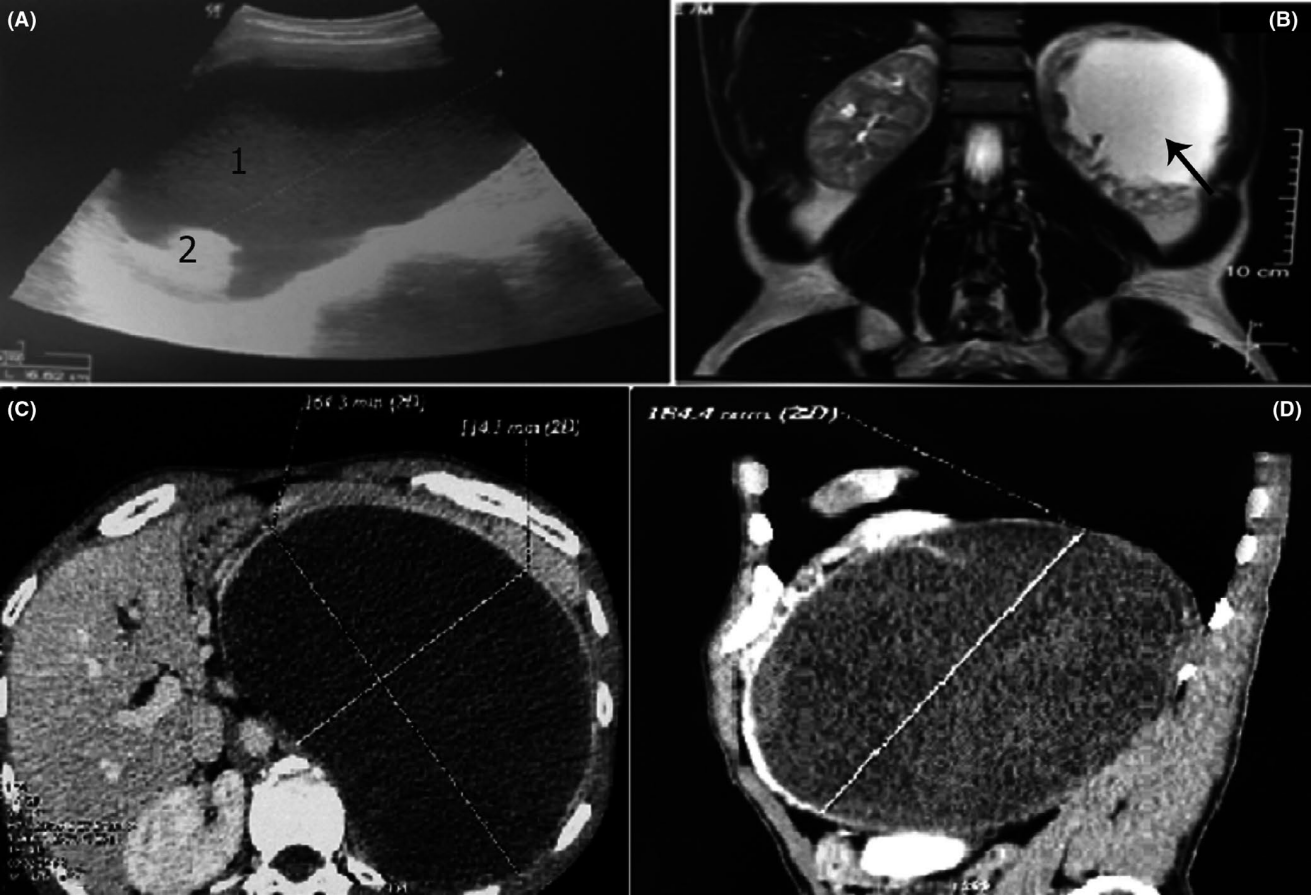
Abdominal imaging showing large adrenal mass in a 46XX patients with congenital adrenal hyperplasia due to 21‐hydroxylase deficiency. Abdominal ultrasound (A) showed left adrenal mass containing mixed cystic (1) and solid (2) components. The mass (arrow) was hyperintense on coronal T2‐weighted MRI (B). It measured 16.4 x 11.4 x 18.4 cm on abdominal CT scan (C‐D)
